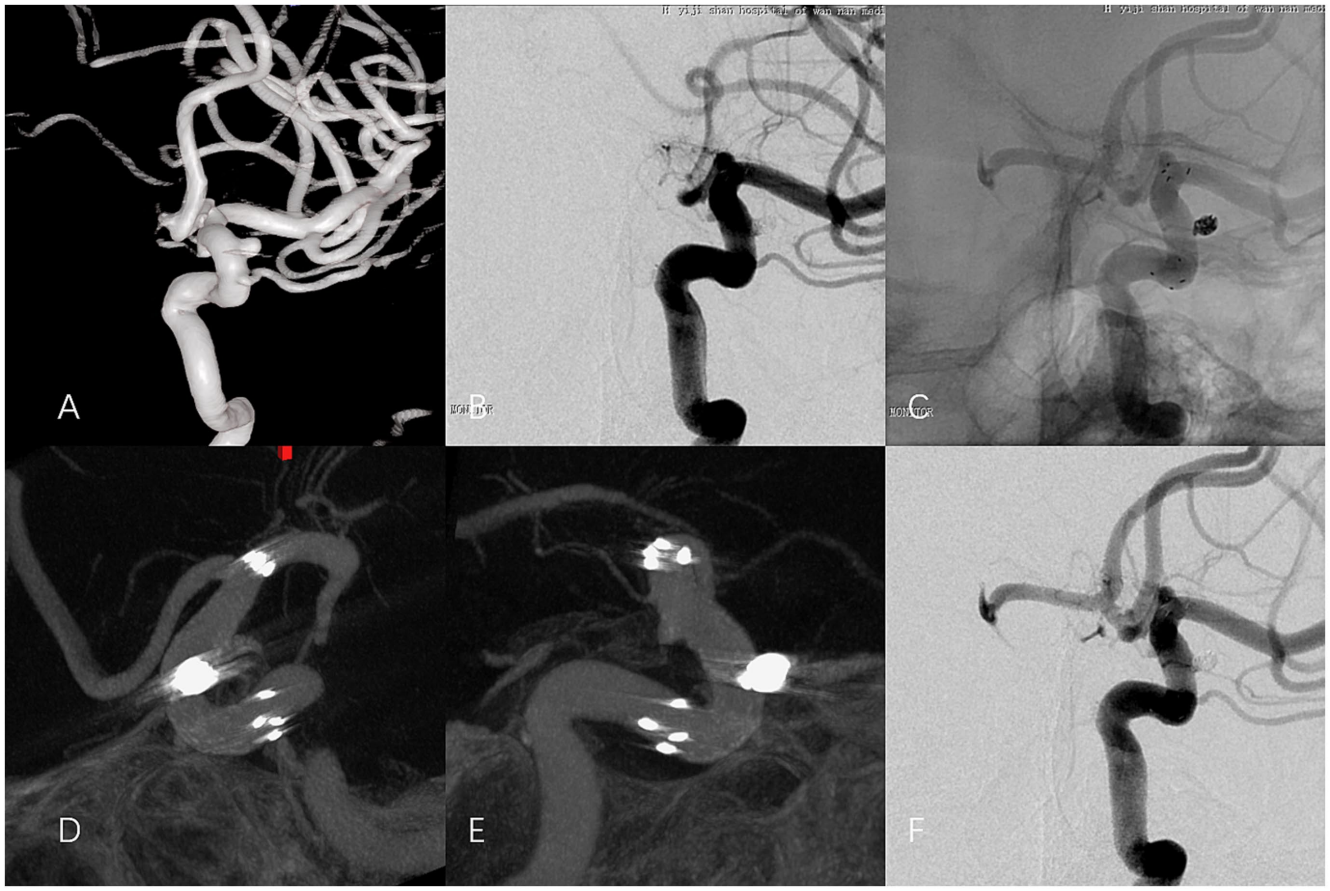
Representative CA images of an unruptured left OSA in a 71-year-old female treated with an EP-VRD2 stent. (A) 3D reconstruction of DSA showing the left OSA. (B, C) Immediate postoperative angiography revealed complete occlusion, with (B) subtraction and (C) un-subtraction images. The stent size was 4.0 × 30 mm. (D, E) Dyna CTA projections showing CA of the stent on both the inner and outer arcs in the curved ICA siphon. (F) DSA at 19 months confirmed complete occlusion.
